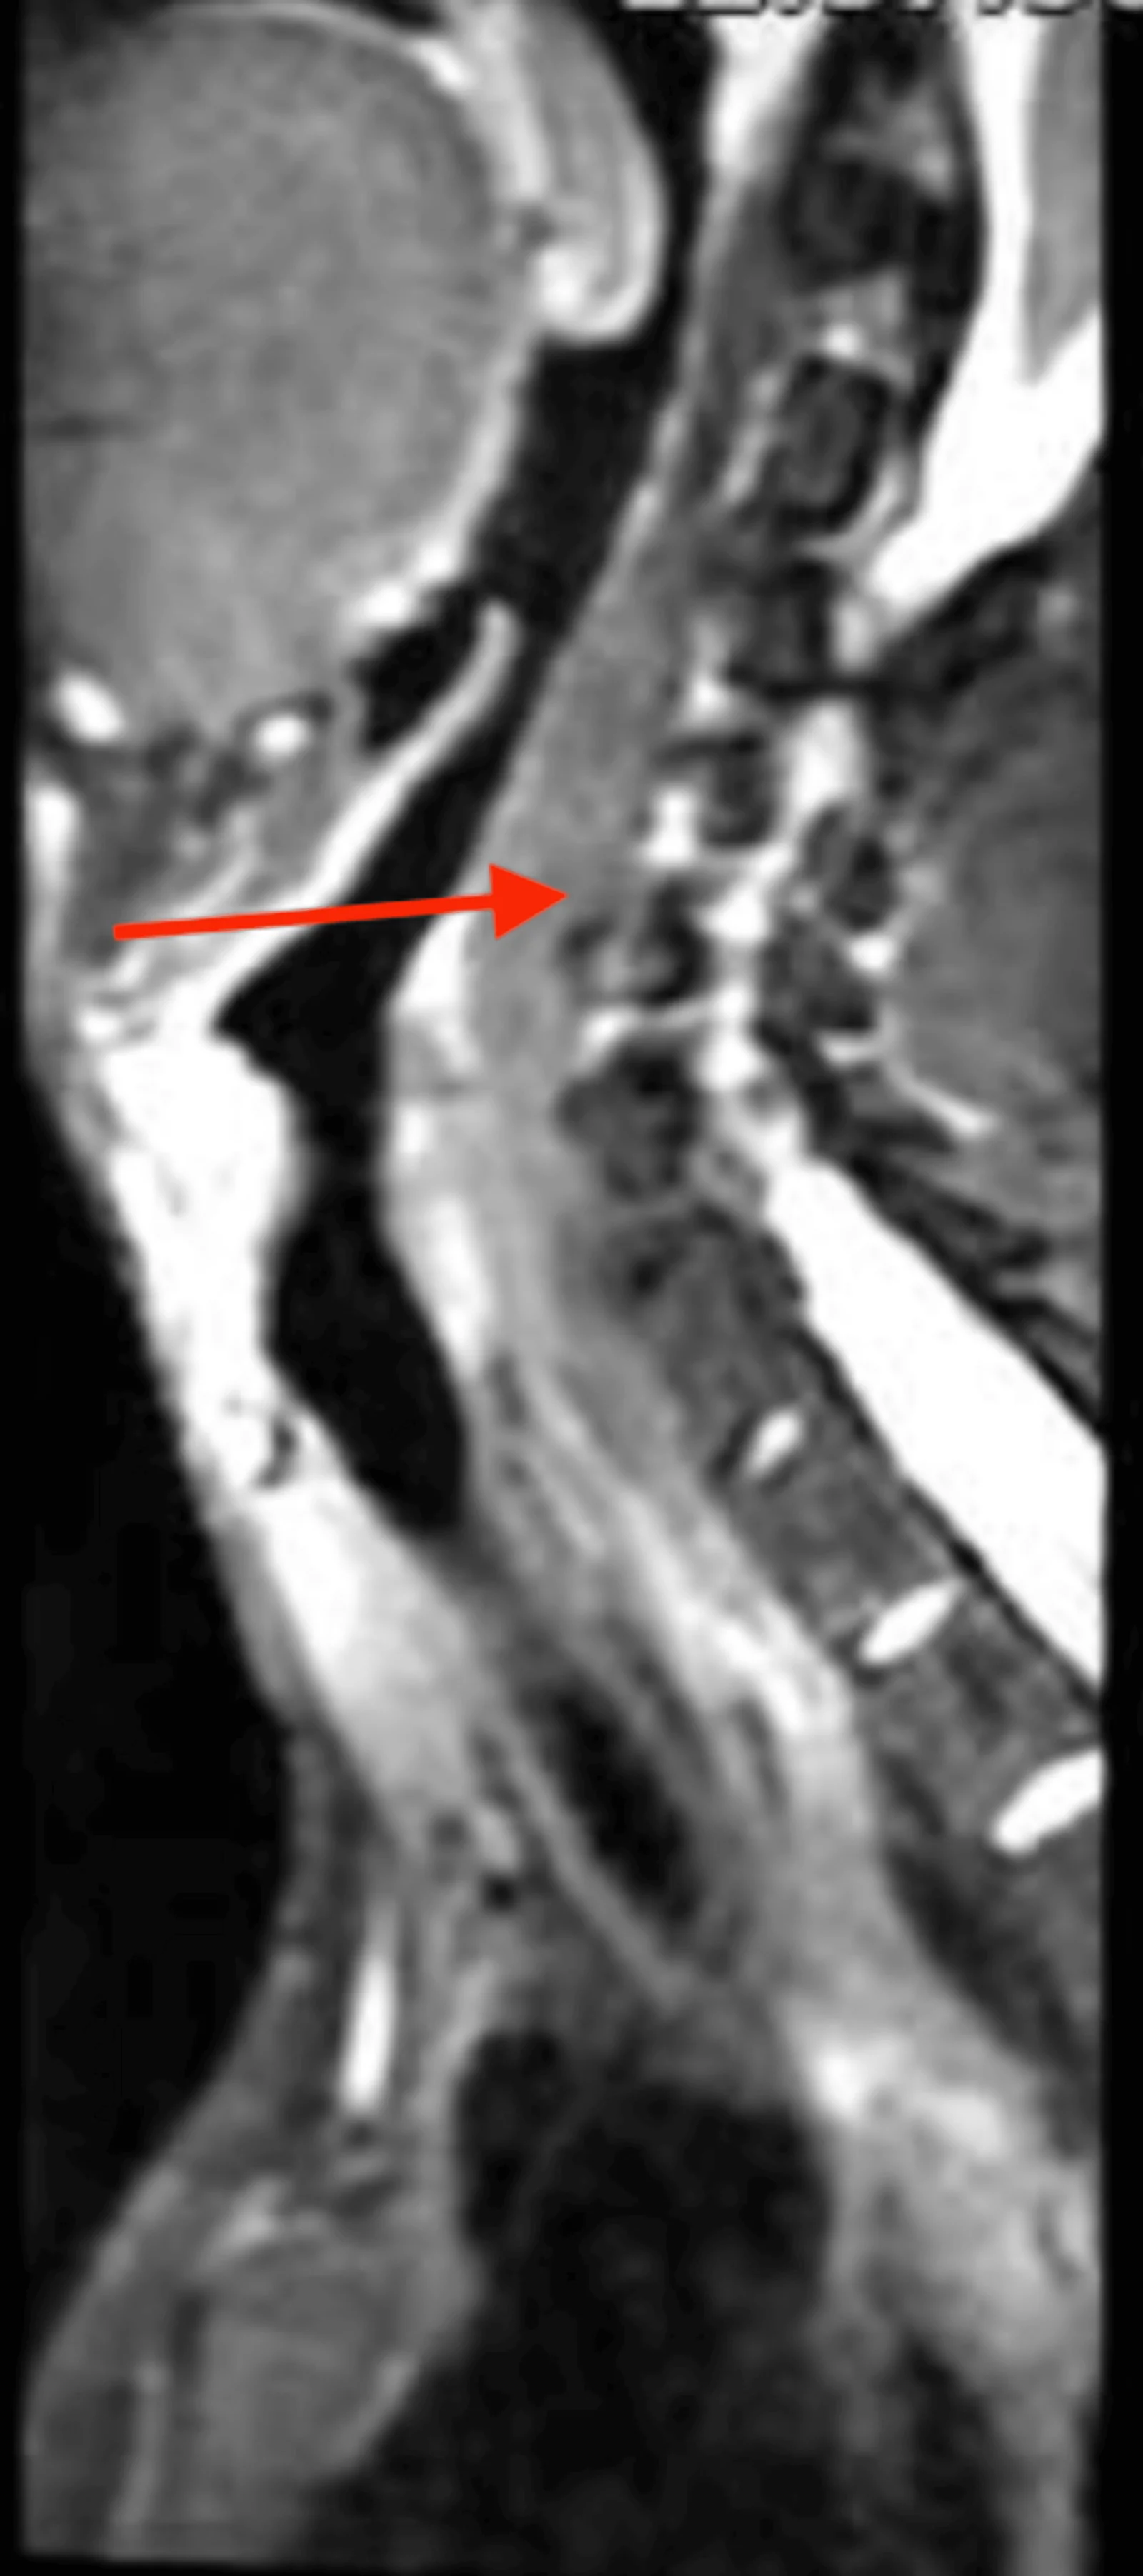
T2 cube sagittal MRI L brachial plexus W/Wo contrast Enhancement, mild thickening, and nodularity of left brachial plexus, most prominently involving the left C5 and C6 nerve roots and left superior trunk.
